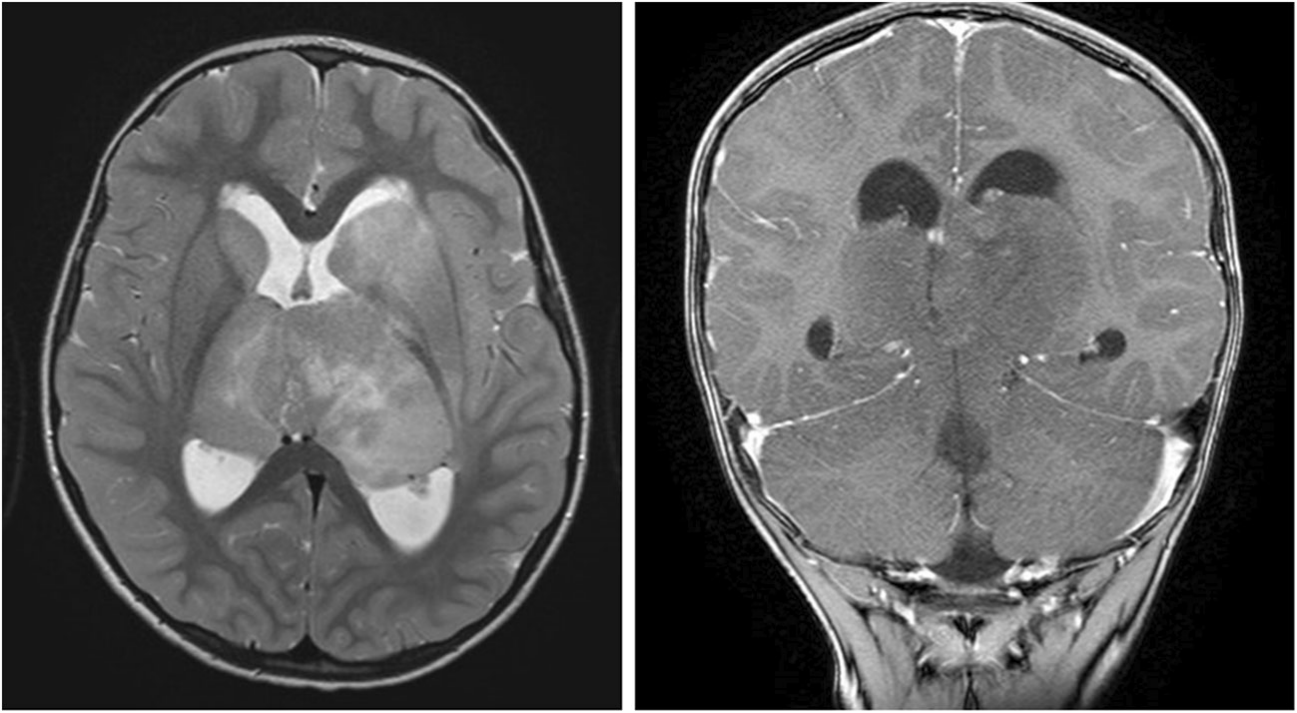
Bilateral thalamic glioma (BTG): axial T2-weighted and coronal T1-contrast-enhanced MRI of a 4-year-old boy presenting with symptoms of increased intracranial pressure. MRI shows a diffuse bithalamic tumor, extending to the basal ganglia (especially on the left). An endoscopic third ventriculostomy and septostomy were performed. Patient succumbed to disease about 1 year later, following oncological treatment
